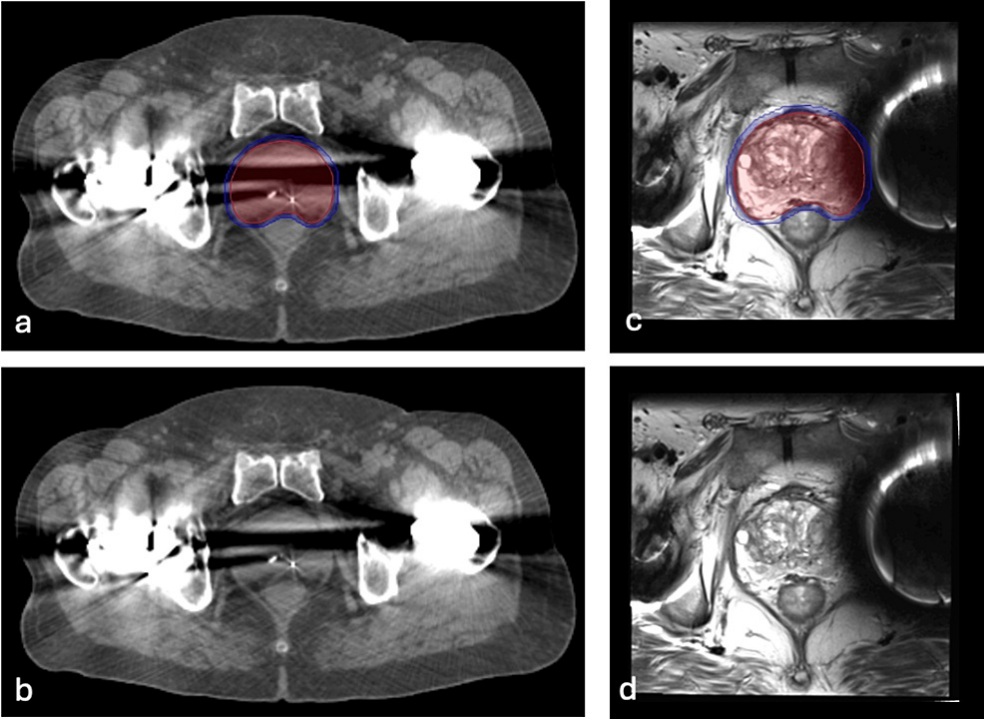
Treatment Planning Images Treatment planning sagittal computed tomography (CT) and magnetic resonance images (MRI) in a 71-year-old man with intermediate-risk prostate cancer and bilateral hip replacements treated with SBRT. The prostate (red) and 100% of the prescription dose (blue) are shown above. (a) CT with contours. (b) CT without contours. (c) MRI with contours. (d) MRI without contours.
